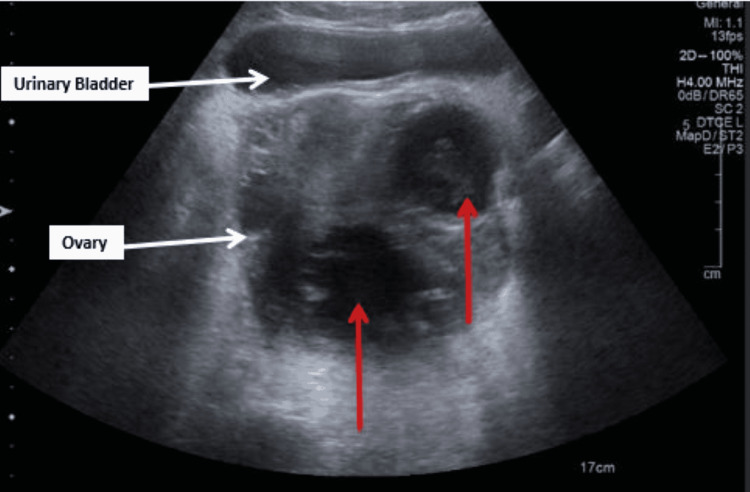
Transvaginal ultrasound image 1 showing 12cm by 6cm and 6cm by 4cm hypoechogenic lesions (indicated by red arrows)
